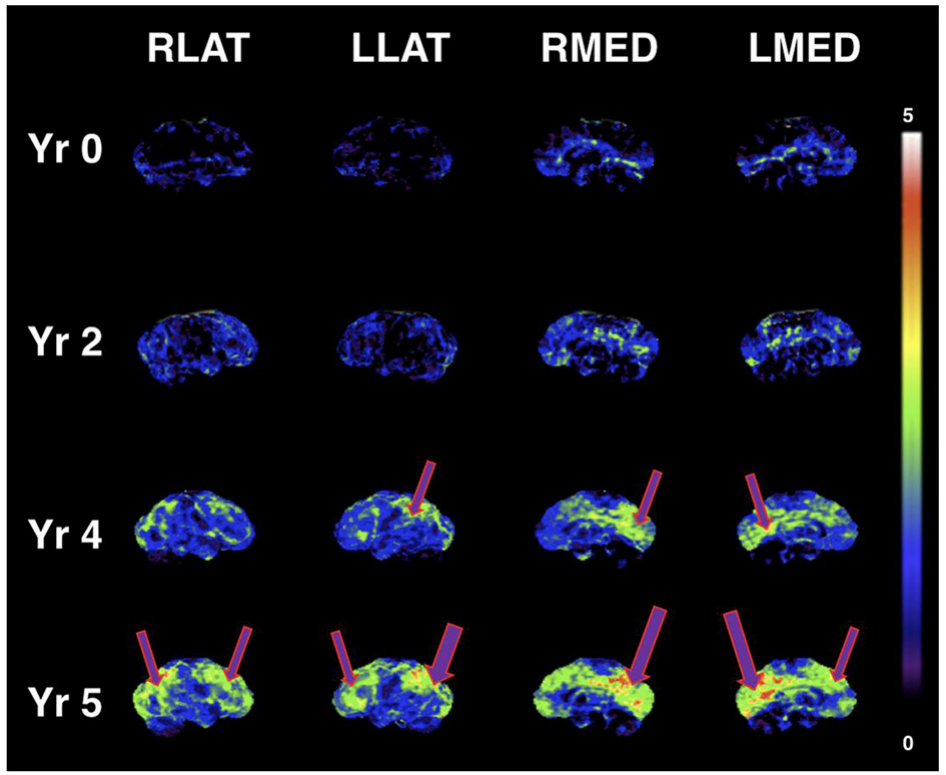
Serial FDG PET images showing progressive metabolic reductions from reference ranges at baseline (Yr 0), through stages of mild cognitive impairment (Yr 2 and Yr 4), and clinical diagnosis of dementia (Yr 5) in PD dementia converter. Cortical Z-score maps based on normal control data are shown (max Z-score is 5; higher scores represent more severe glucose metabolic deficits). Purple arrows represent reduced glucose metabolism. Similar to worsening metabolic deficits seen in MCI and AD, cortical glucose metabolic reductions become more severe and extensive with progression of cognitive decline (in this case, glucose uptake in key brain regions such as the posterior cingulum and precuneus is ~3–5 standard deviations lower than control data at Yr 5). According to the “alternative fuel” hypothesis, escalating dose or potency of ketogenic interventions may be needed for greater brain metabolic deficits (greater brain energy “gap”). Figure reproduced with permission from Bohnen et al. (54).
